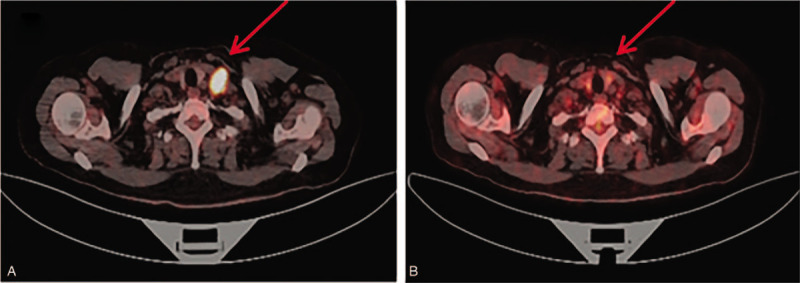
PET-CT showed lymph node metastasis in the left supraclavicular fossa. (A) Before treatment with pembrolizumab and nab-paclitaxel, the metastasis was 1.6 cm in short diameter. (B) After 6 cycles of pembrolizumab and nab-paclitaxel, the metastasis was nearly dismissed. PET-CT = positron emission tomography-computed tomography.
